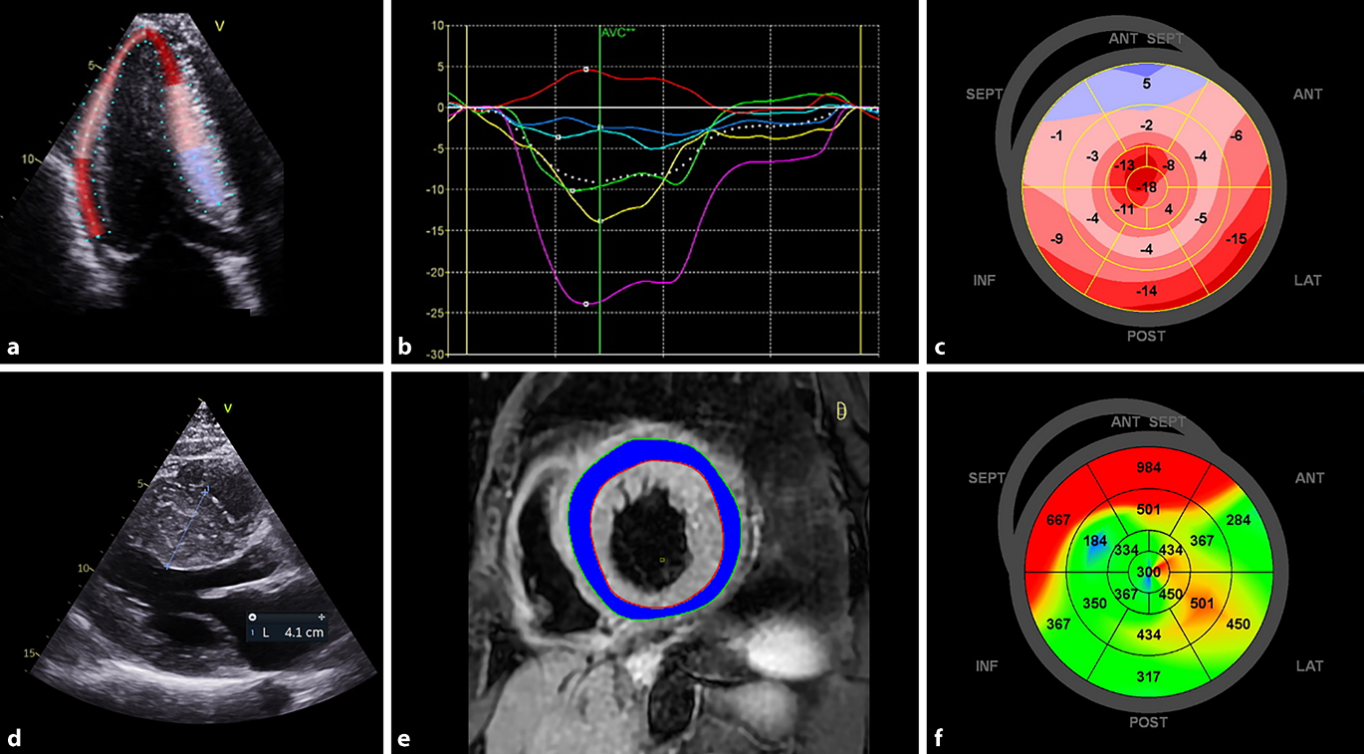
Multimodality imaging to assess the risk of sudden cardiac death (SCD) in hypertrophic cardiomyopathy (HCM). Longitudinal strain is measured with two-dimensional speckle-tracking echocardiography in an apical three-chamber view (a), generating segmental strain-versus-time curves (b). Impairment of left ventricular global longitudinal strain (−8.7%) is shown on a parametric map that demonstrates most severe impairment in the septum (c), which is the area of maximal wall thickening (d). T2-weighted short-tau inversion recovery short-axis image of the left ventricle, demonstrating an elevated T2 signal intensity ratio (3.3) when left ventricular myocardium (*blue*) is compared to skeletal muscle (*yellow*) (e). Mechanical dispersion (MD) of the left ventricle in the same patient as in a–d, displayed as a parametric map (f). Late activation of the septal segments (*orange/red*) results in an abnormally elevated MD of 183 ms (f). Impaired left ventricular global longitudinal strain, increased T2 signal intensity and increased MD are all associated with a higher SCD risk in HCM. *ANT* anterior, *AVC* aortic valve closure, *INF* inferior, *LAT* lateral, *POST* posterior, *SEPT* septal
